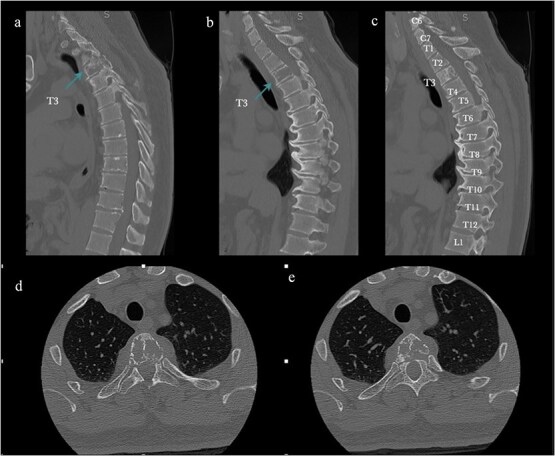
Preoperative CT. (a–c) Sagittal bone window: Osteolytic T3 vertebral body lesion. (d, e) Axial views: Vertebral body compromise. The arrow shows the T3 level.
